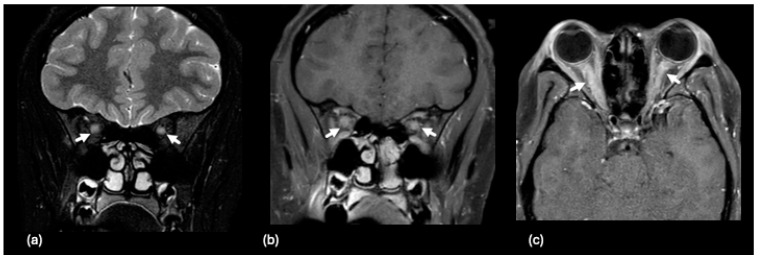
A 13-year-old female presenting with bilateral papilledema and imaging findings of optic neuritis. Coronal T2-weighted image (a) with fat saturation demonstrates bilateral optic nerve edema and swelling (white arrows). Coronal (b) and axial (c) fat-saturated, post-contrast T1-weighted images demonstrate abnormal enhancement (white arrows). The authors formally obtained permission to use clinically generated medical images stored within the Cincinnati Children’s Hospital Medical Center’s PACS for this figure on 6 December 2024.
